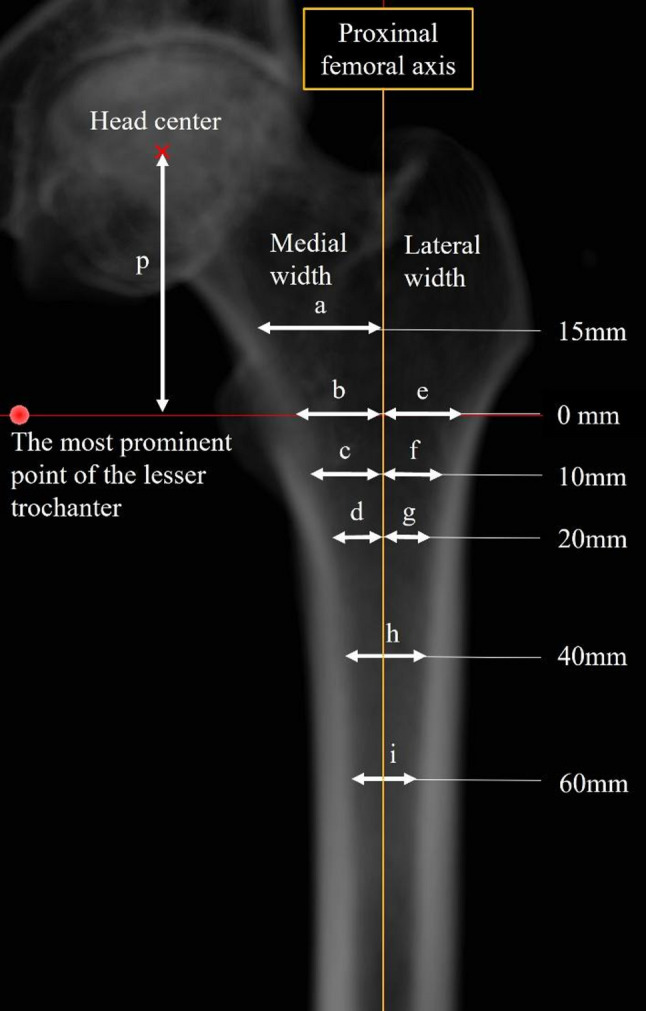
Projection images are created using three-dimensional (3D) computed tomography, and the femoral dimensions are measured at 10 points based on the proximal femoral axis and the most prominent point of the lesser trochanter, which are used as features
